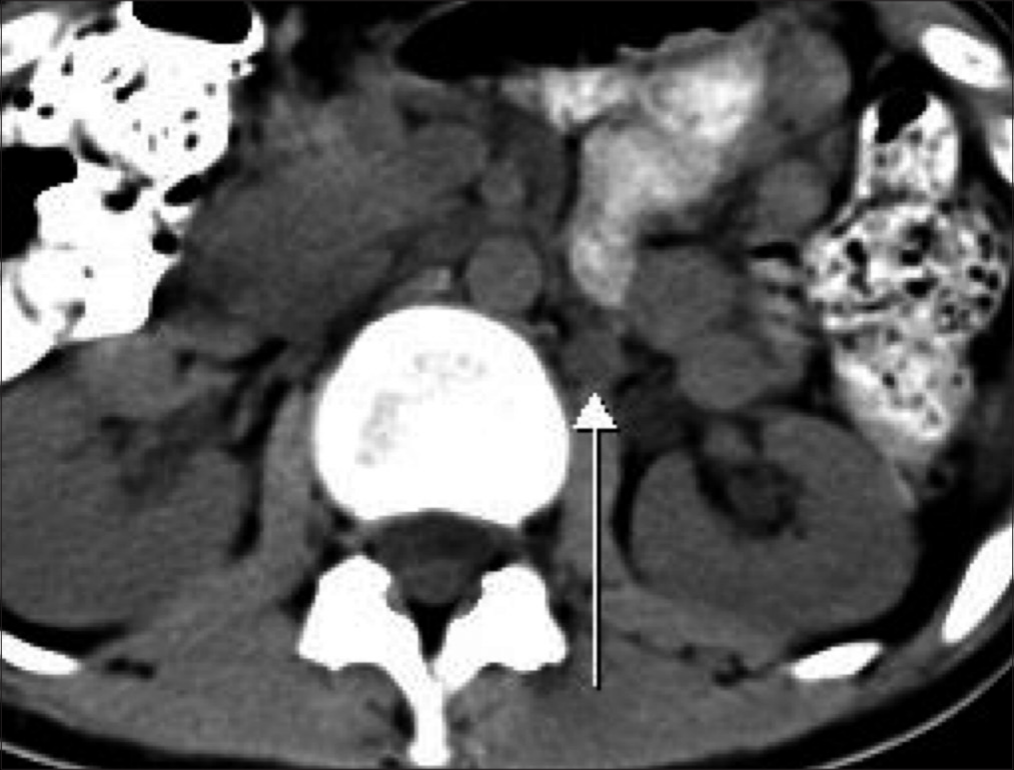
Axial nonenhanced CT scan shows a well-defined round structure (arrow) adjacent to the left renal hilum
